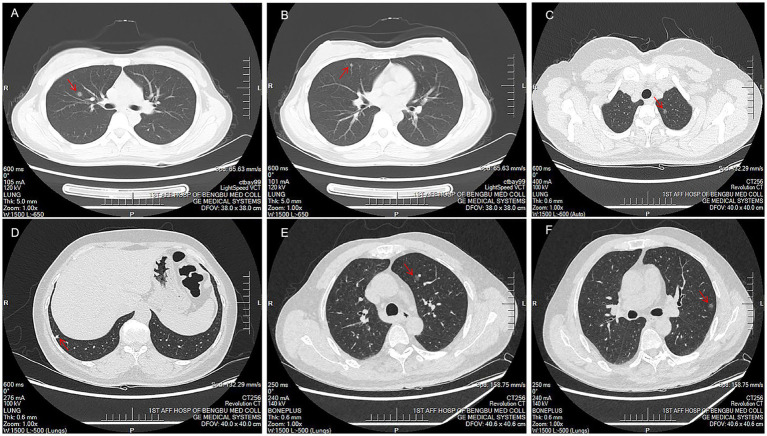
Ground-glass nodules of pulmonary sclerosing pneumocytoma (PSP) on CT. (A, B) A patient with PSP coexisting with lung adenocarcinoma: (A) A ground-glass nodule with blurred margins is seen in the anterior segment of the right upper lobe. (B) A ground-glass nodule is present in the middle lobe of the right lung. (C, D) Another patient: (C) A ground-glass nodule is observed in the apicoposterior segment of the left upper lobe. (D) A ground-glass nodule is present in the basal segment of the right lower lobe. (E, F) Another patient: Ground-glass nodules are observed in both the anterior and apicoposterior segments of the left upper lobe.
